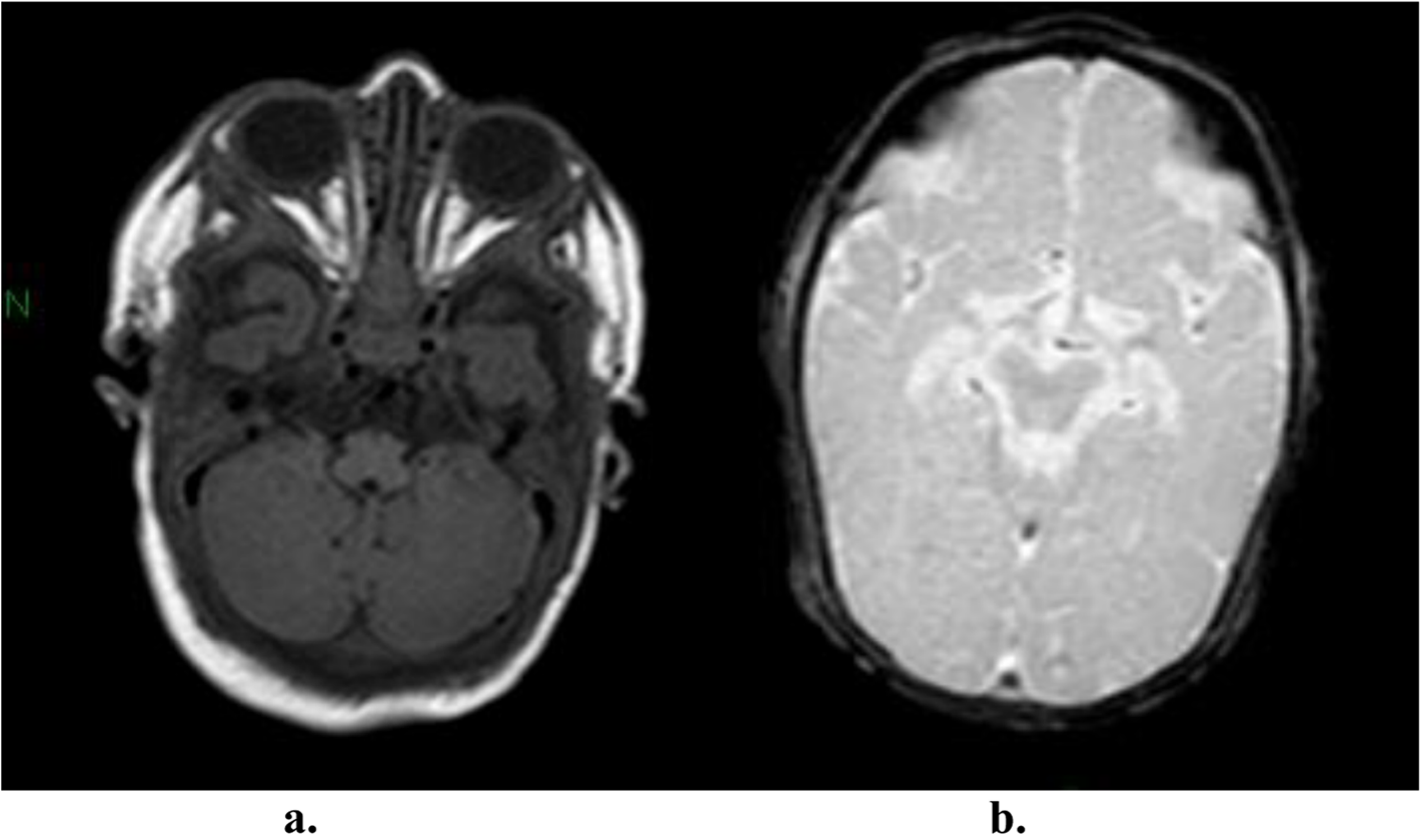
a-b Brain MRI performed at 3 months: a T1 coronal sequence showing simplified cortical structures (shallow sulci); b T2 coronal sequence showing micro cavitation localized in the pre and post rolandic subcortical white matter areas
